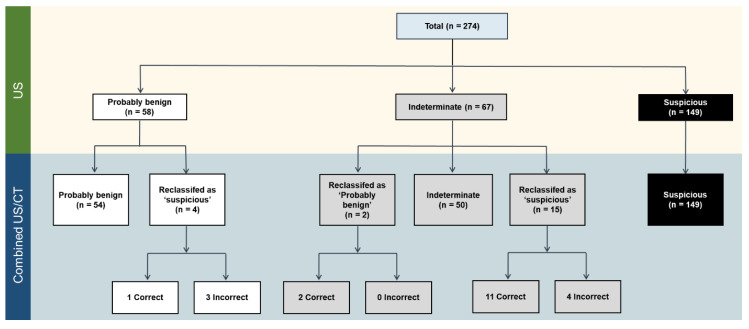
Changes in the LN classification with the addition of CT. CT—computed tomography, LN—lymph node, US—ultrasonography.
